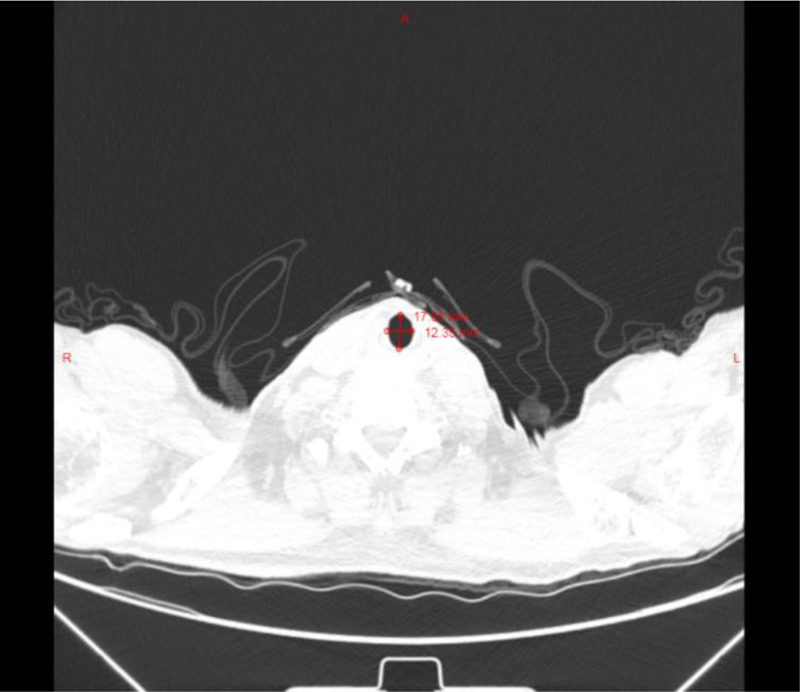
Chest CT imaging demonstrated no significant dilation of the subglottic trachea, with sagittal and coronal diameters measuring 17.05 and 12.35 mm, respectively. CT = computed tomography.
